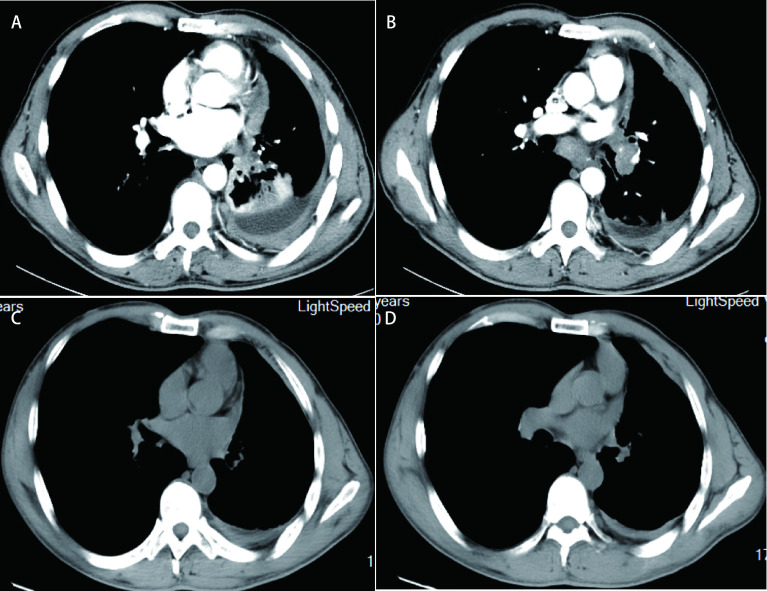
克唑替尼治疗前后CT图像。A：克唑替尼治疗前CT图像(2016年3月24日)：左侧包裹性胸腔积液; B：克唑替尼治疗前CT图像(2016年3月24日)：纵隔淋巴结肿大，左肺下叶占位(2.6 cm×1.4 cm); C：克唑替尼治疗后CT图像(2019年6月25日)：左侧包裹性胸腔积液较前减少; D：克唑替尼治疗后CT图像(2019年6月25日)：纵隔淋巴结肿大较前缩小，左肺下叶小病灶(1.3 cm×0.6 cm)缩小。 CT images before and after crizotinib treatment. A: CT images before crizotinib treatment (24-Mar-2016): left encapsulated pleural effusion; B: CT images before crizotinib treatment (24-Mar-2016): mediastinal lymphadenopathy, left lung lower lobe space occupying (2.6 cm×1.4 cm); C: CT images after crizotinib treatment (25-Jun-2019): left encapsulated pleural effusion was reduced; D: CT images after crizotinib treatment (25-Jun-2019): mediastinal lymph node enlargement was reduced, small lesion in the lower lobe of the left lung (1.3 cm×0.6 cm) was reduced.
